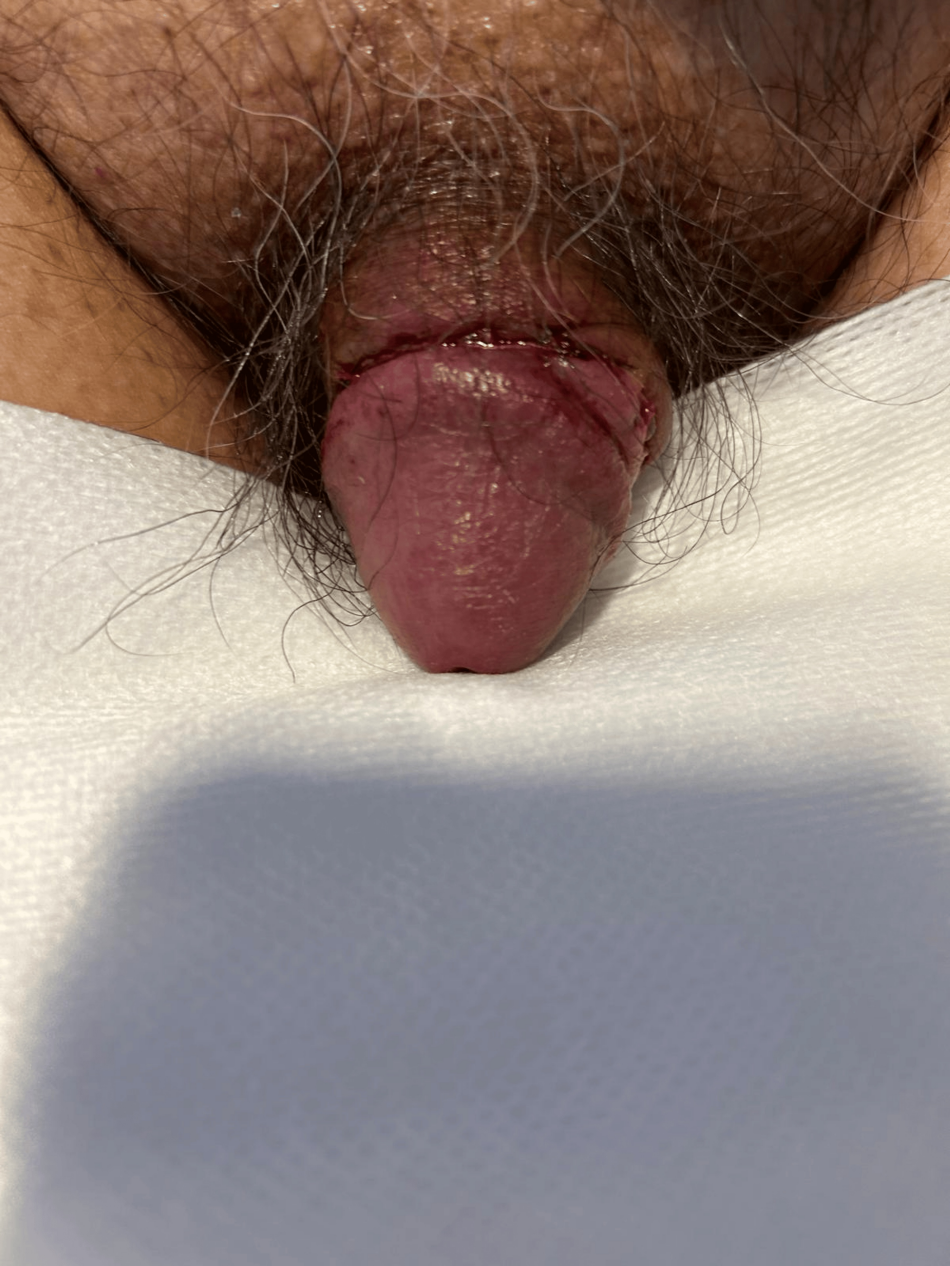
Day 1 postoperative image of penis post circumcision using an ultrasonic dissecting scalpel. There is no postoperative bleeding or oedema of the penis.
